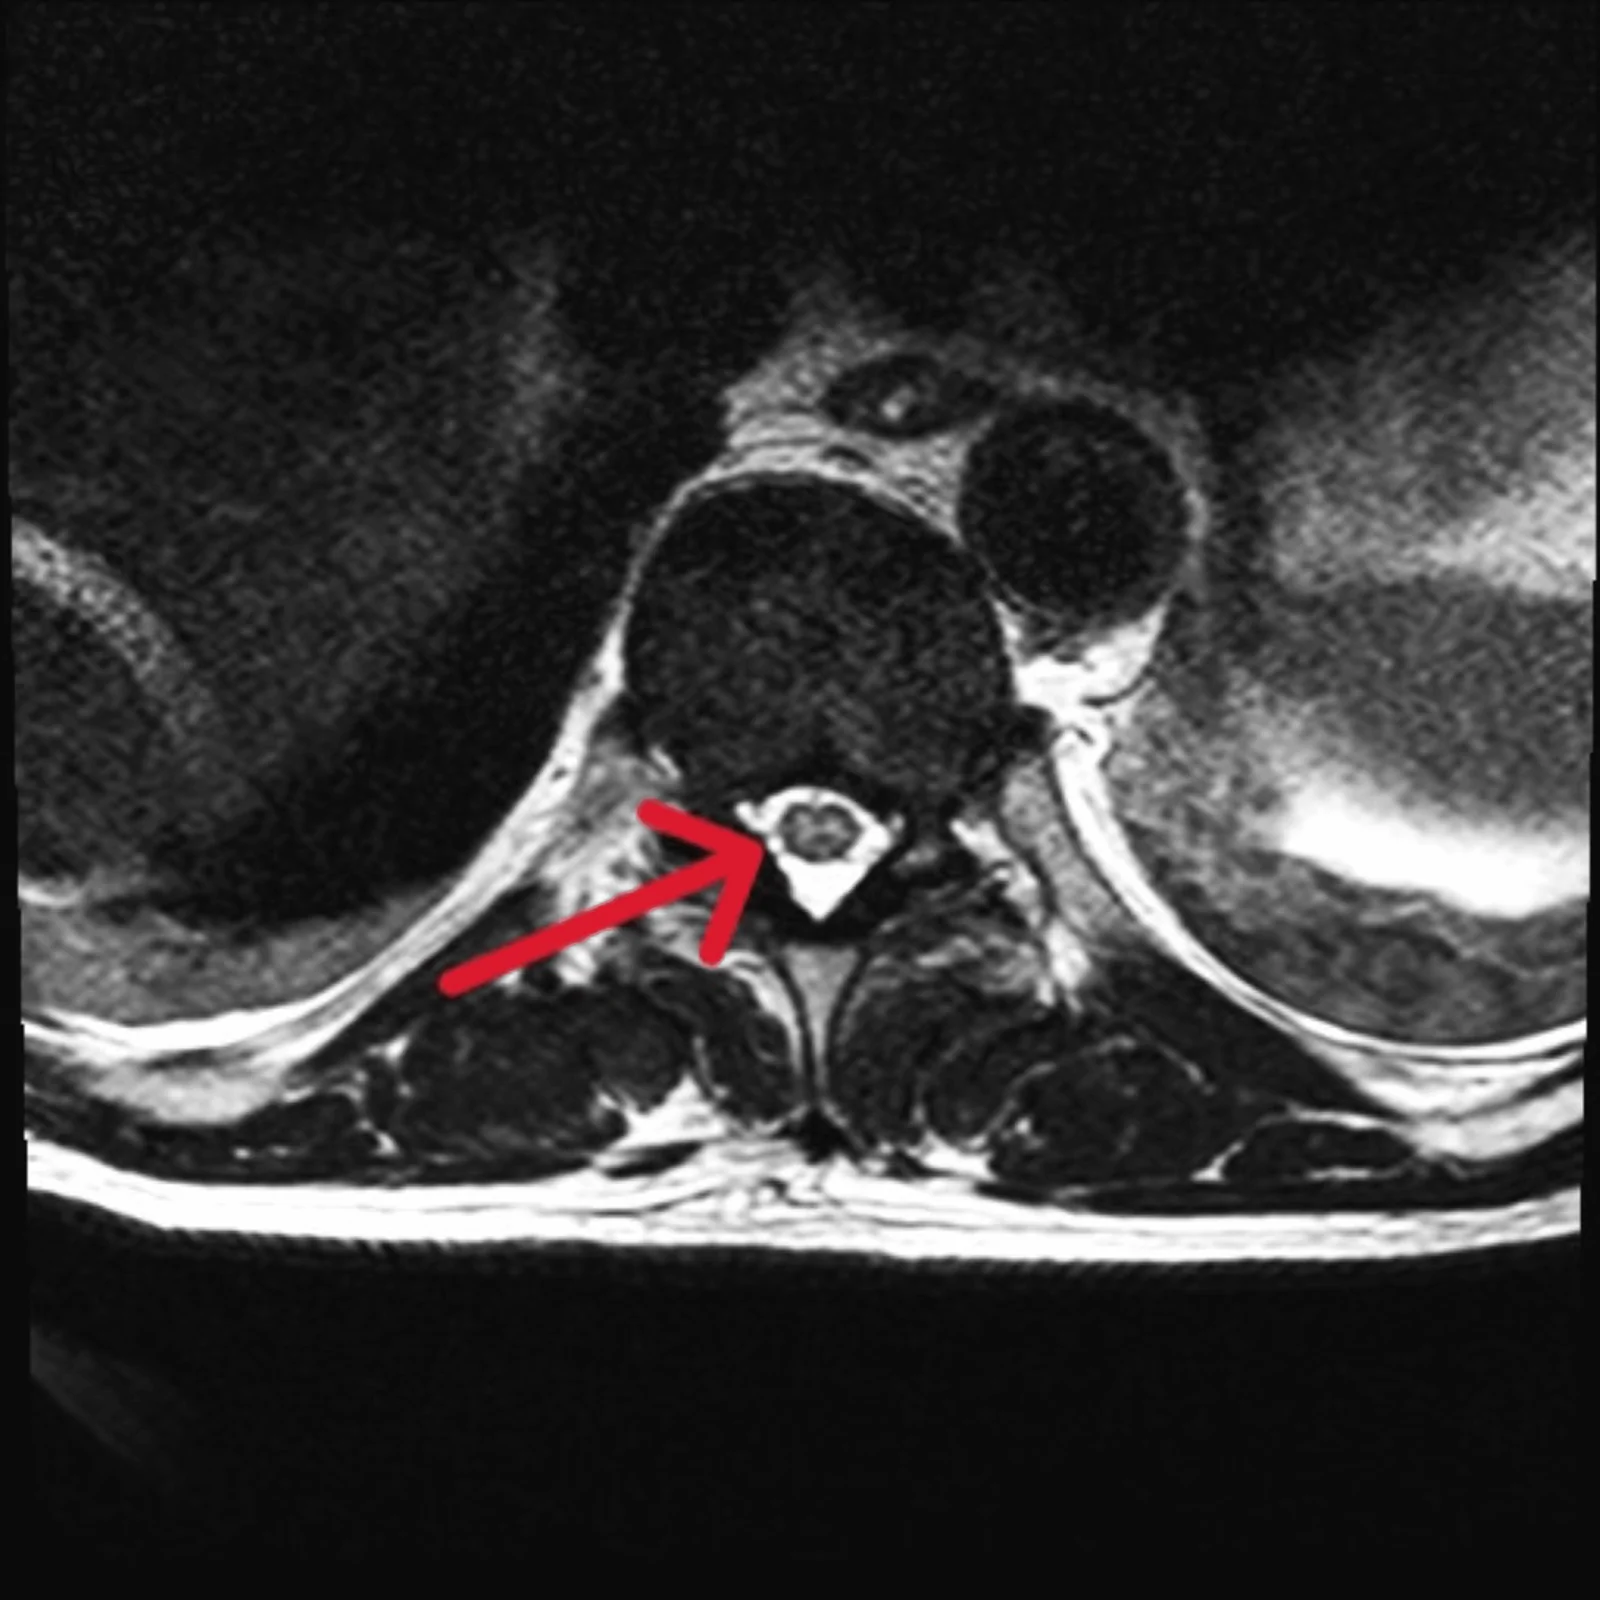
MRI findings of acute flaccid myelitis Spinal MRI demonstrates focal T2 hyperintensity involving the anterior gray matter/anterior horn region, supporting acute flaccid myelitis with anterior horn cell involvement. Adapted from Sapkota P [11]. West Nile virus encephalomyelitis. Radiopaedia.org case (rID: 174050), doi:10.53347/rID-174050, accessed June 1, 2026, used in accordance with the applicable Radiopaedia Creative Commons license.
